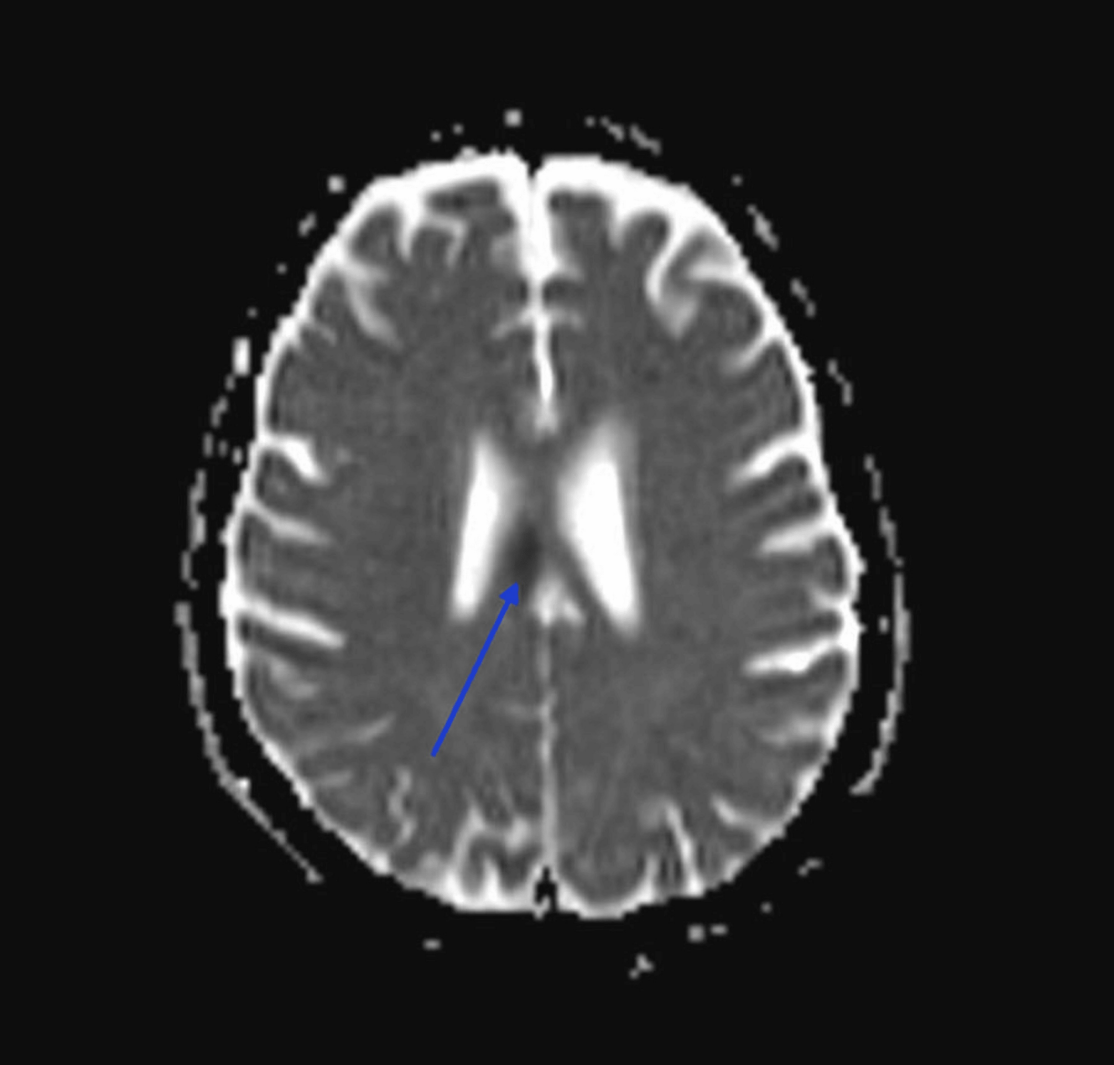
MRI brain, axial view, ADC sequence, showing hypointensity in the right body of the corpus callosum. ADC: apparent diffusion coefficient.
